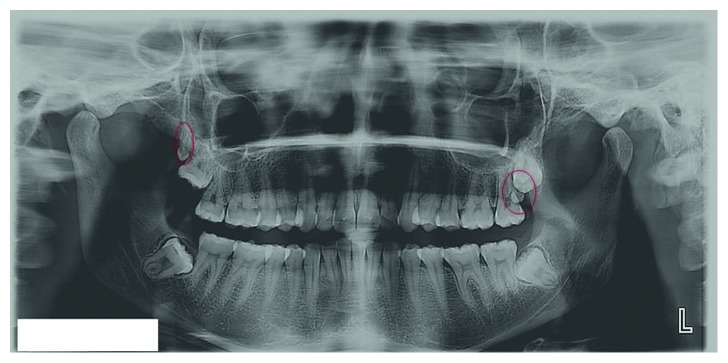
Panoramic radiograph showing maxillary the right distomolar and left paramolar (encircled).
